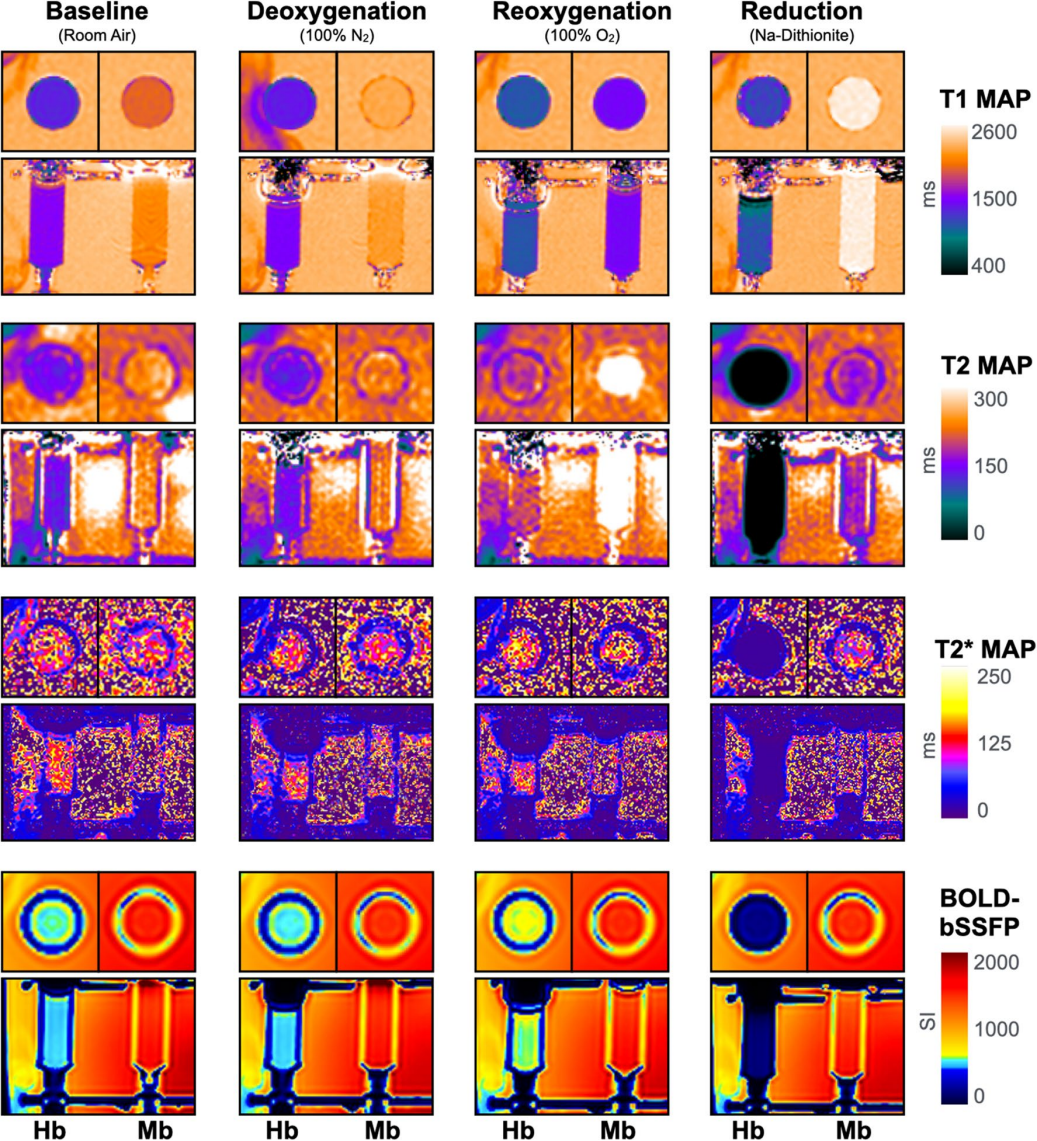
MRI imaging of myoglobin and hemoglobin: Horizontal and vertical cross-section views are shown of representative T1 maps, T2 maps, T2* maps and blood oxygen level-dependent balanced steady state free precession (BOLD-bSSFP) of red blood cells and dissolved myoglobin in modified test tubes at each major oxygenation state. On the left side a hemoglobin sample is shown, while on the right side a myoglobin sample is shown within the same imaging plane and for all acquired imaging sequences, respectively. Image prepared using Circle cvi^42^ version 5.13 (www.circlecvi.com).
